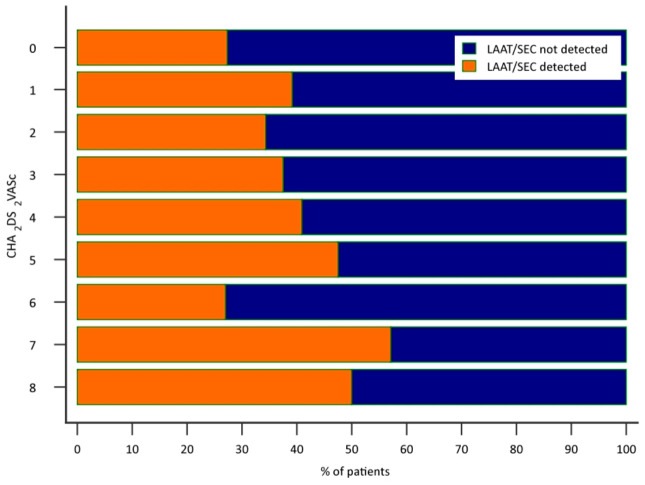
Left atrial appendage thrombus and/or spontaneous echocardiographic contrast detection distribution at different CHA_2_DS_2_-VASc score levels.
